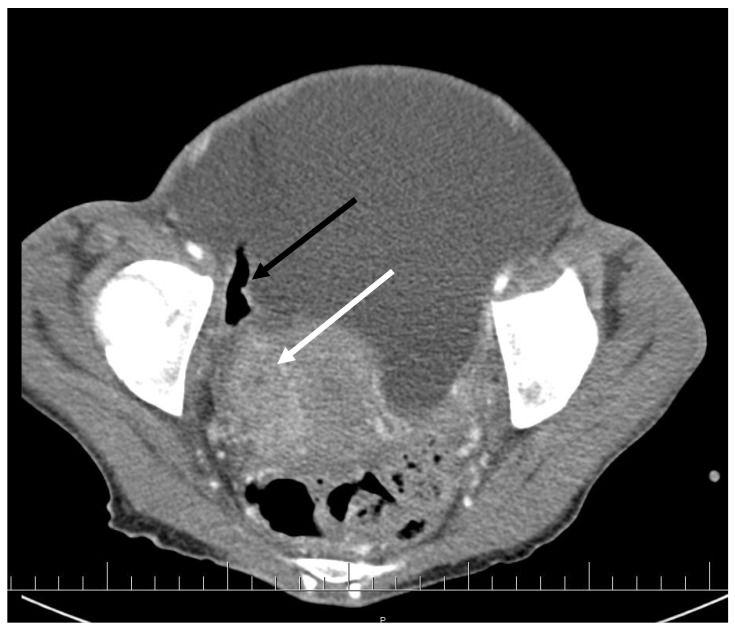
Pelvic CT scan shows ascites, enlarged right ovary (white arrow) and a gas-containing non-homogenous mass adhering to the uterus (black arrow).
